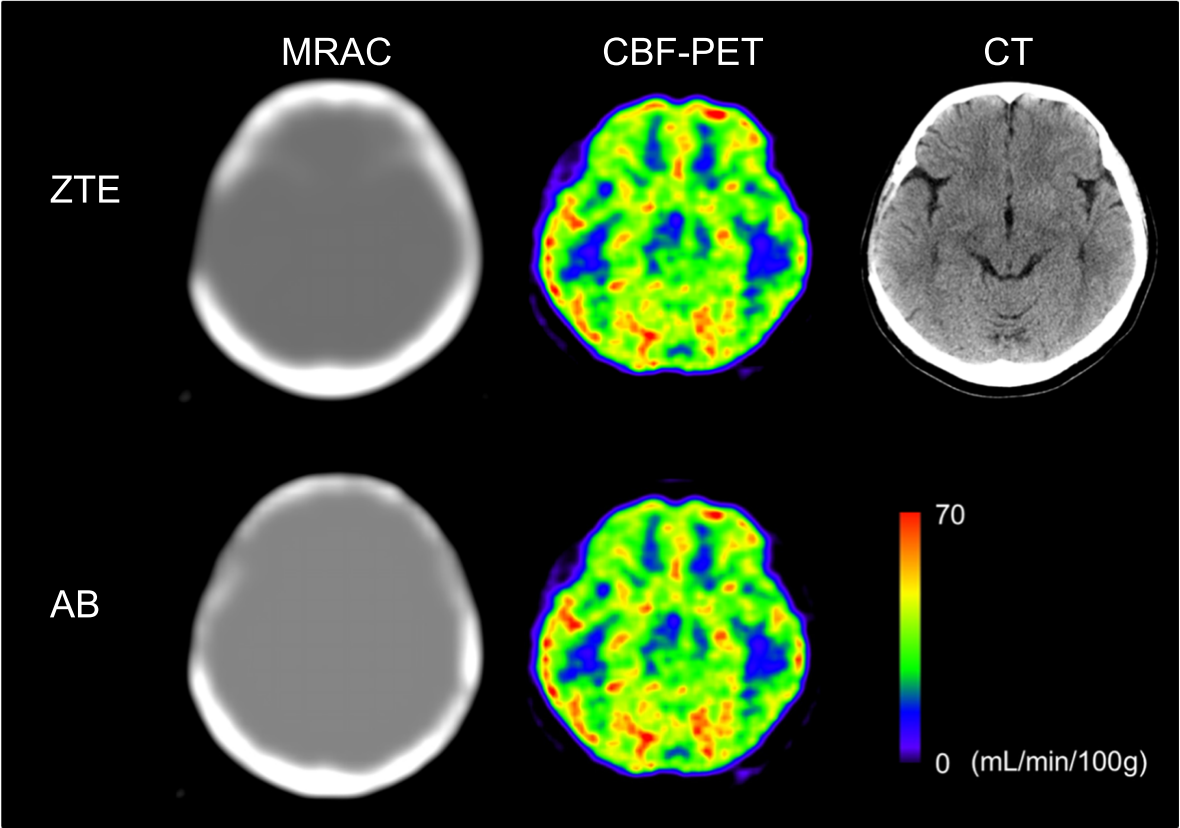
ZTA-, AB-MRAC, and CBF images at the same slice level from a patient who underwent neurosurgical treatment. Top row shows the ZTE-MRAC, CBF, and CT at the corresponding slice level (right). The defect of the right temporal bone is clearly delineated by the ZTE-MRAC (left). The bottom row shows the images from the AB-MRAC method (left), which generated the right temporal bone. CBF images from the two methods show that CBF values in the right temporal lobe were very close (middle column). This PET scan was not included in the analysis
